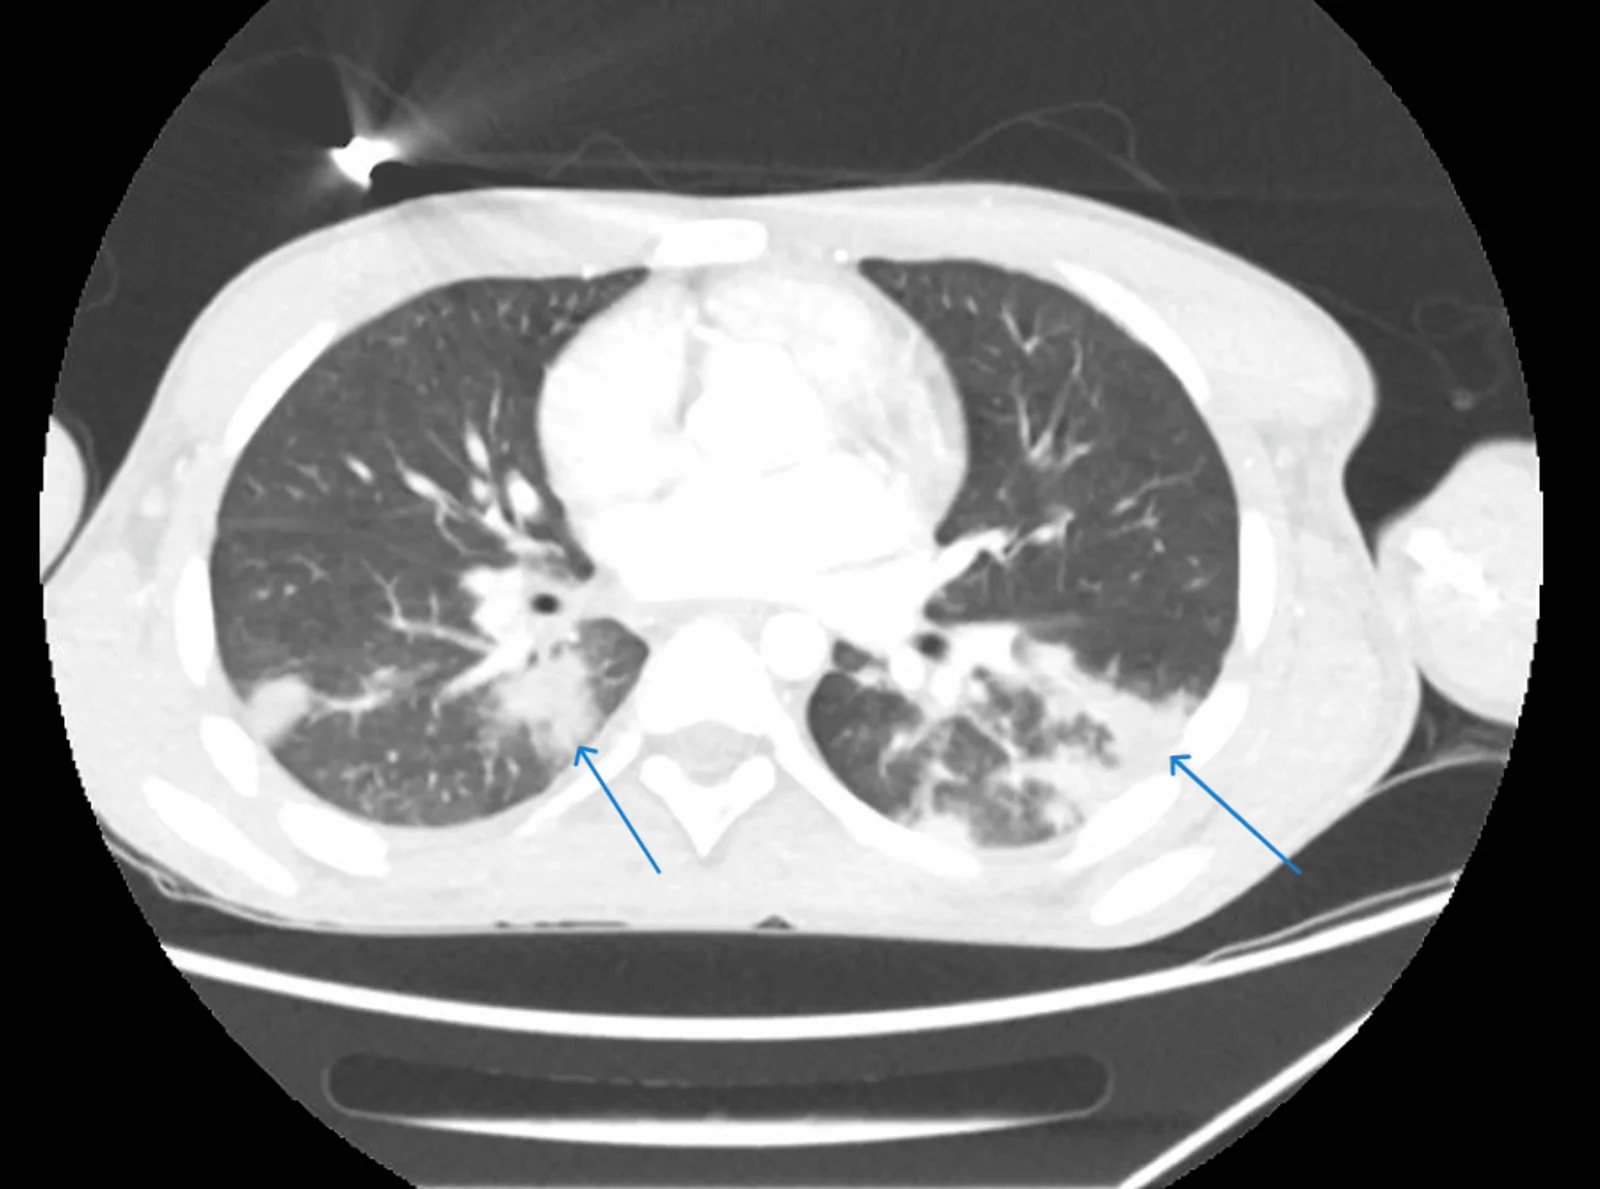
Computed tomography (CT) imaging of the lung parenchyma demonstrating bilateral patchy consolidation involving the right and left lower lobes (blue arrows) suggestive of septic embolisation.
